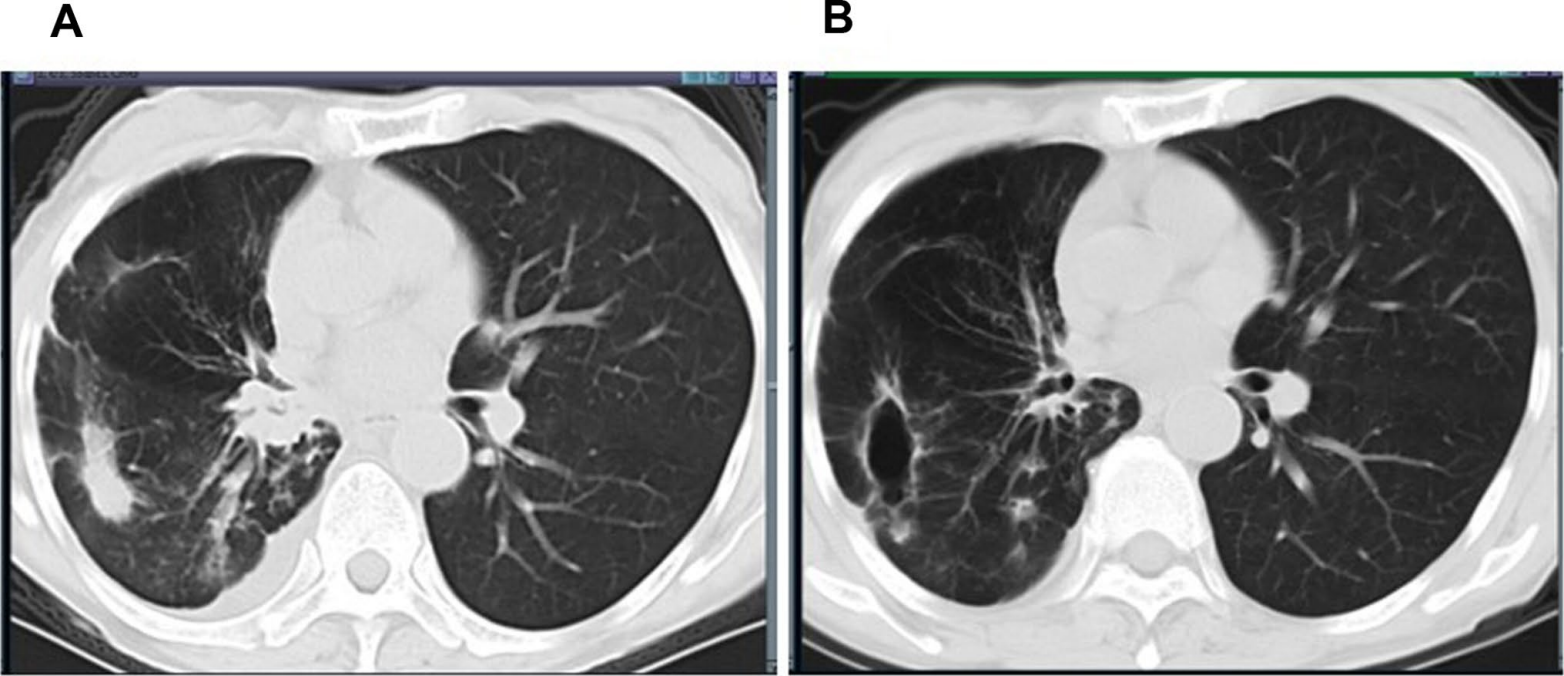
Chest CT scan showed an almost complete tumor response after three cycles of durvalumab treatment. A Before durvalumab treatment. CT plane scan revealed right lower lobe peripheral lung cancer with hilar lymph node metastasis. Simultaneously, CT contrast enhanced imges showed right lower pulmonary artery invasion, right lower pulmonary vein and right atrium tumor thrombus, right adrenal metastasis. B After durvalumab treatment for three cycles. CT lung window revealed Focal necrosis, thin-walled cavity formationthe hilar lymph nodes were significantly reduced. The morphology of hilum of lung returned to normal
